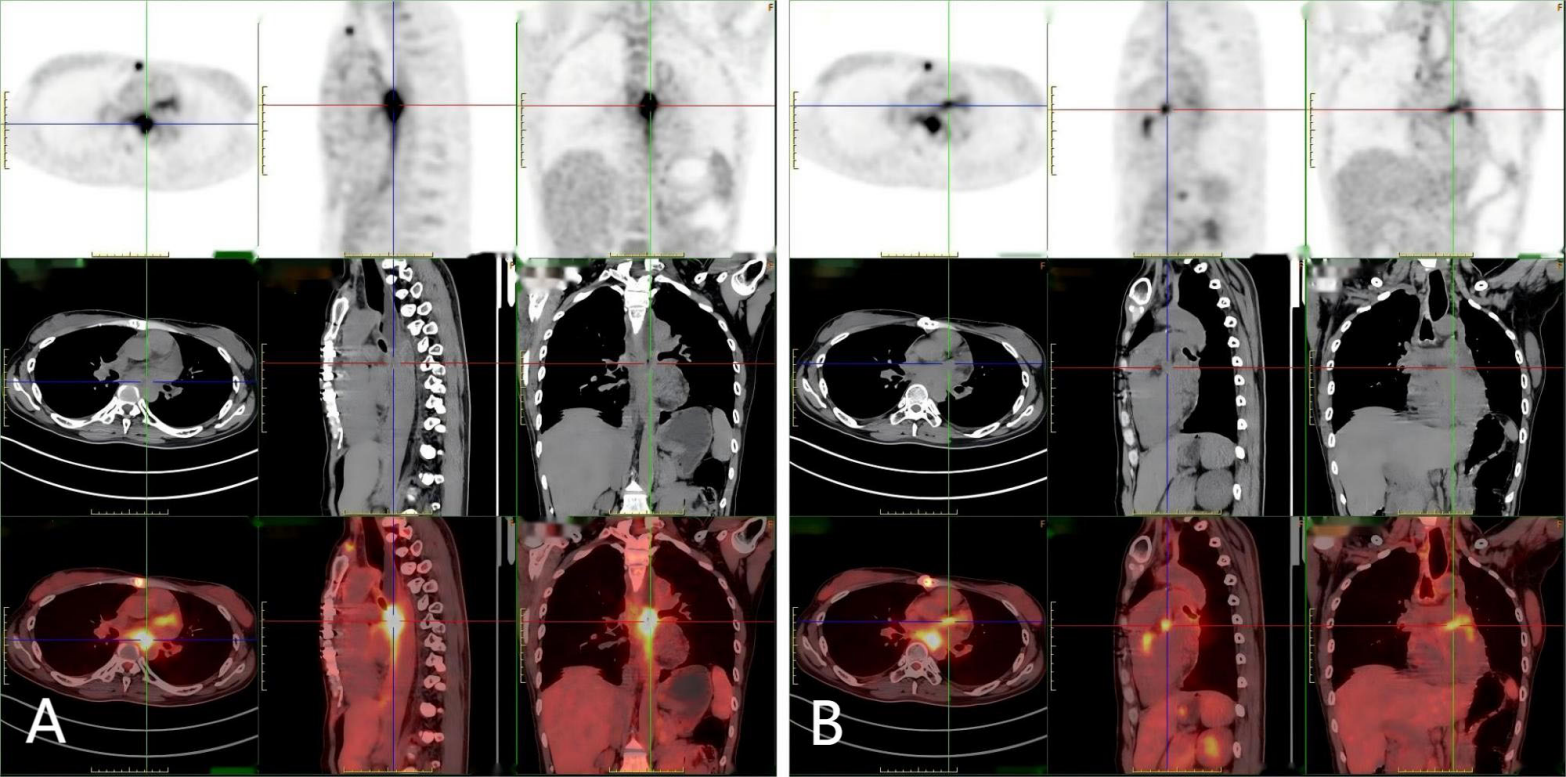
(A, B) A ^18^F-FDG-PET-CT scan: Hypermetabolic lesion in the left atrium wall and esophagus.
